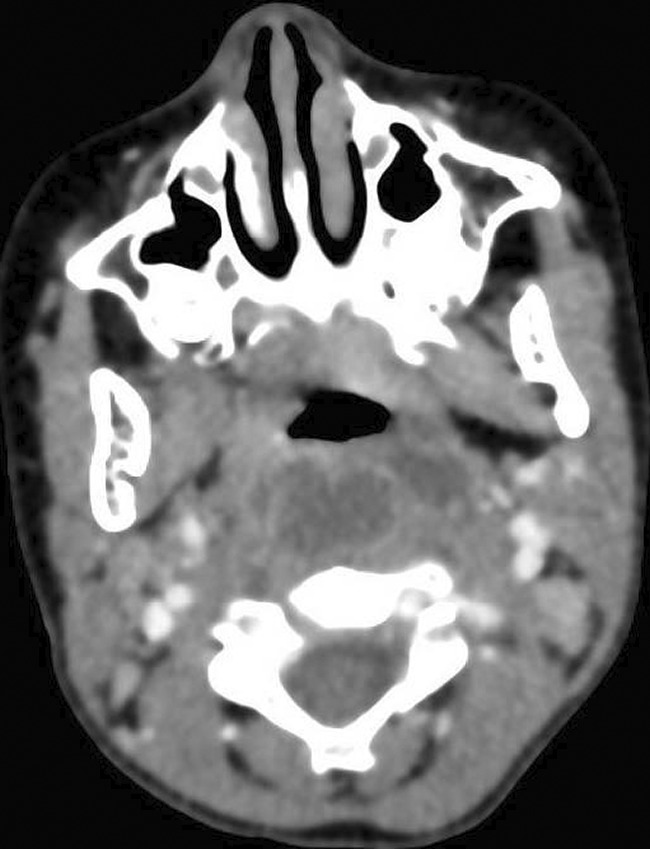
CT head & neck with contrast revealed well‐defined retropharyngeal abscess measuring 3.7 × 1.5 × 2.1 cm
